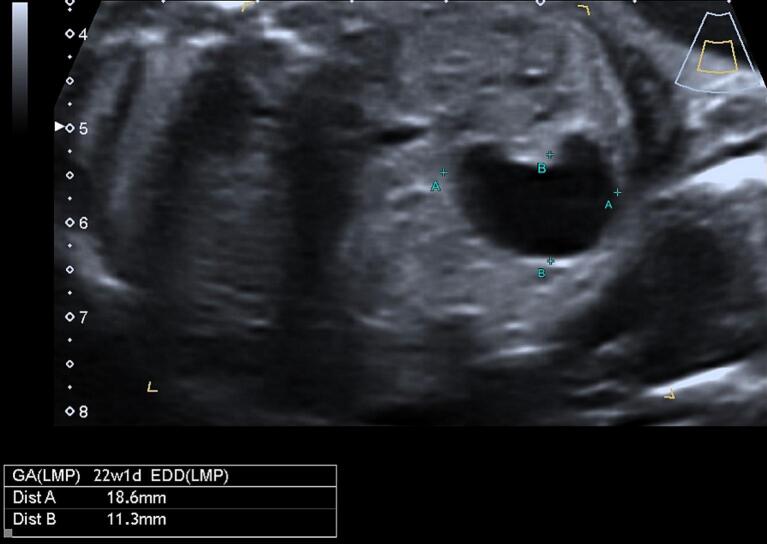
Ultrasound unilocular abdominal cystic mass image at 22 weeks of gestation.
